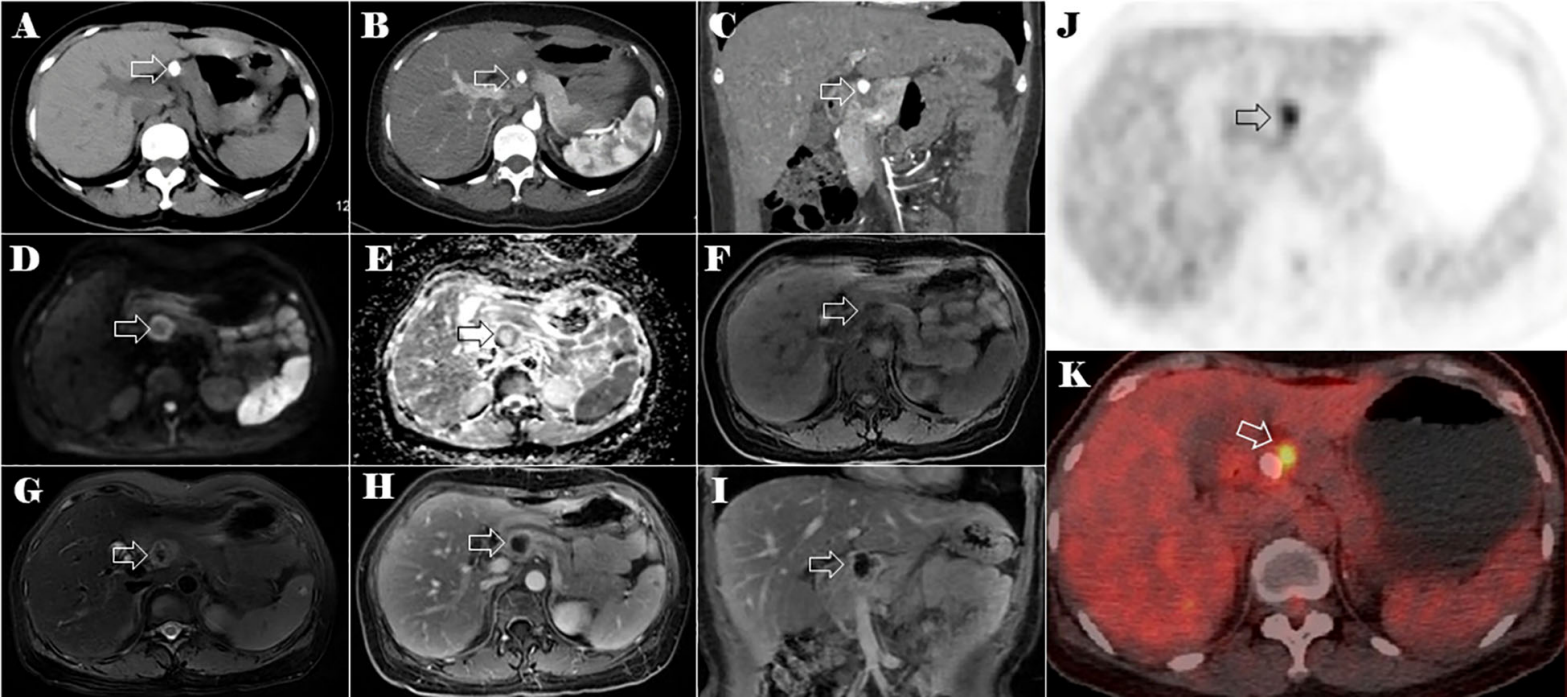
CT, MRI, and PET-CT images of the lesion in the neck of the pancreas (the lesion is indicated by the hollow arrow). Plain CT axial image (A), arterial phase of contrast-enhanced CT axial (B) and coronal (C) images of the abdomen. The axial multi-sequence MRI images of the pancreas on DWI (D), a ADC* image (E), a T1-weighted image (F), a T2-weighted fat-suppression image (G), and a T1 enhanced image (H). Coronal T1 enhanced image (I) of the lesion in the neck of the pancreas. Axial PET-CT images of the lesions in the neck of the pancreas (J, K).
